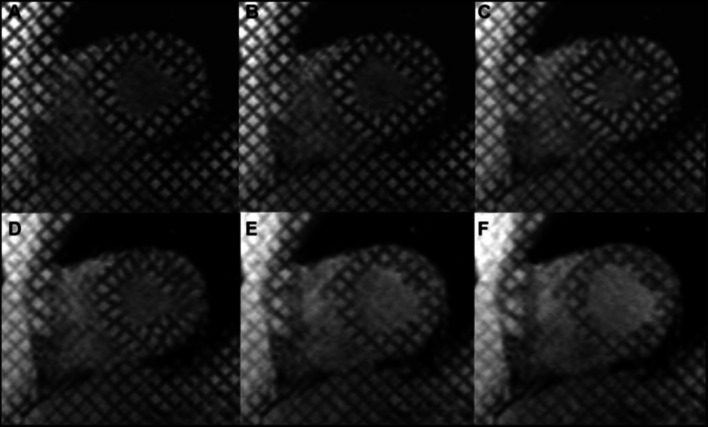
Short-axis tagged MRI mid-cavity slices: a tagging produced at end-diastole; b–d tag lines deform with myocardial contraction in systole; e, f tag lines deform with myocardial relaxation in diastole; f tag lines fade as the end of a complete cycle is approaching [24]
